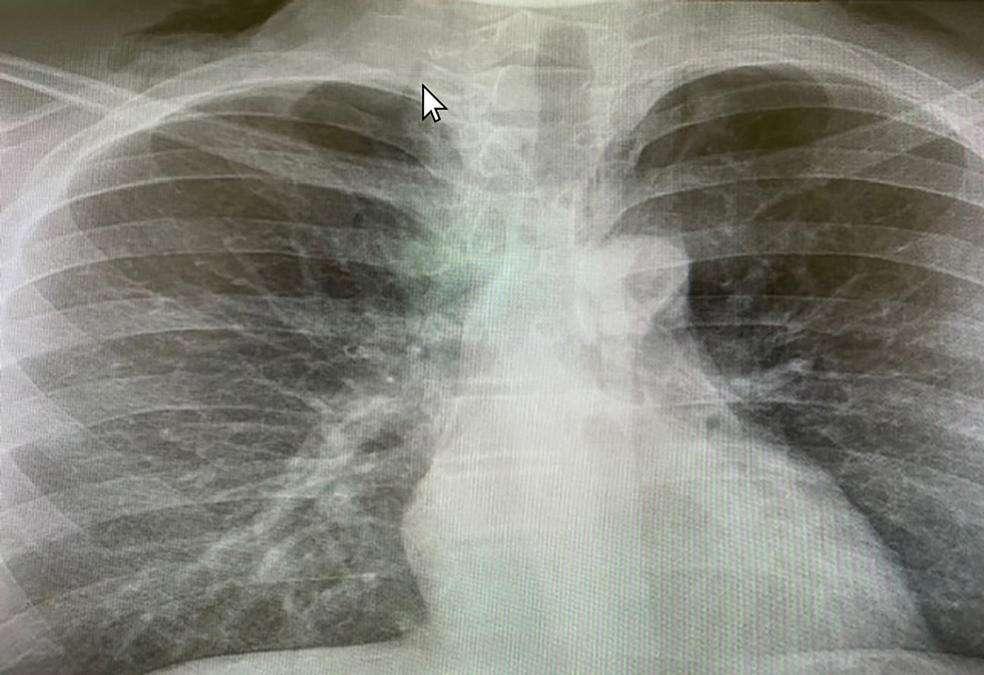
Plain X-ray of a posteroanterior view showing a bilateral lower neck and soft tissue subcutaneous emphysema. The arrow points to a gaseous streak seen centrally at the root of the neck.
